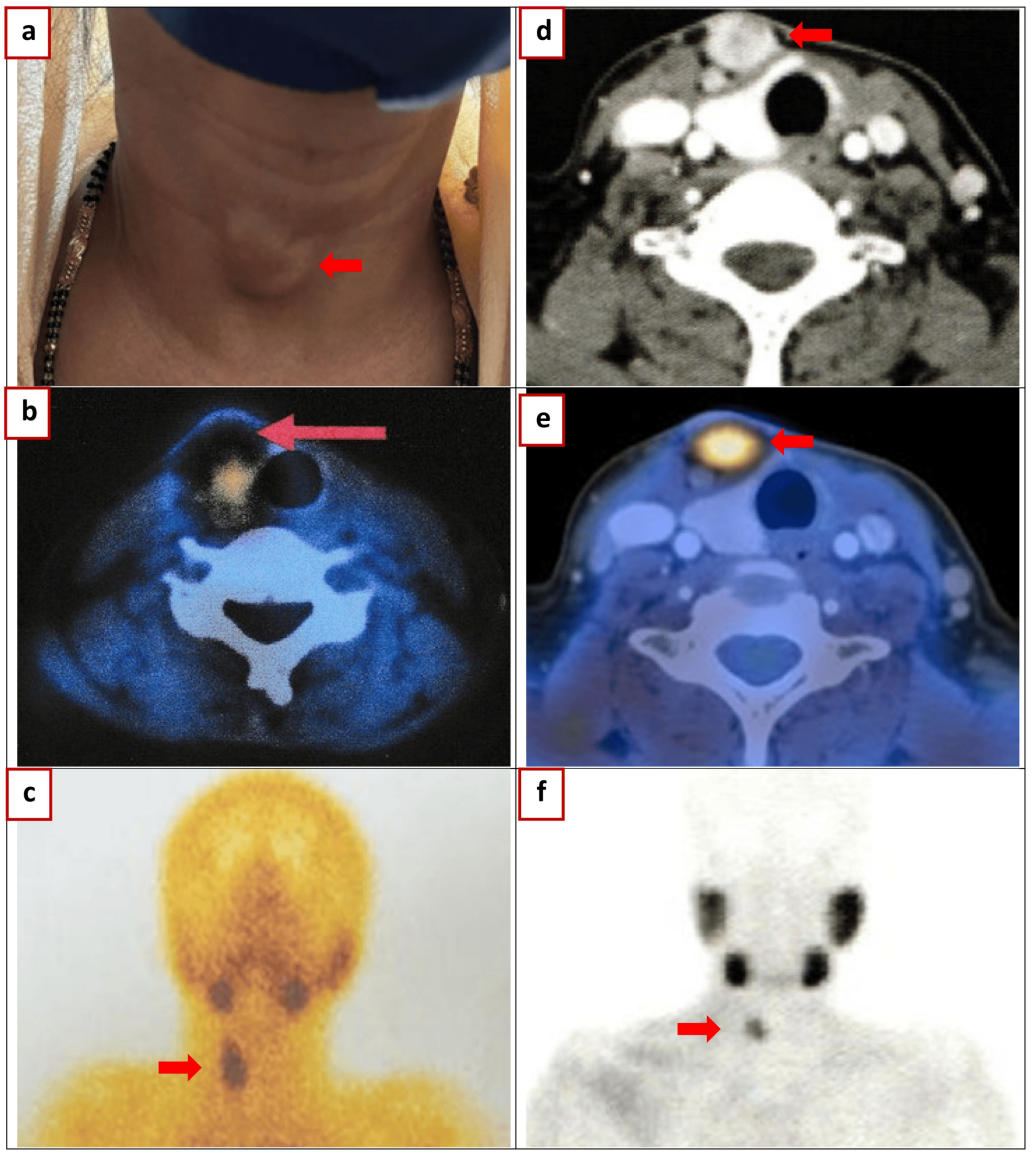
Nuclear medicine imaging (a-c) 2023 January: Technetium (Tc) 99m sestamibi single photon emission computed tomography (SPECT) CT scan: Subcutaneous cystic lesion anterior to the right lobe of the thyroid. No other evidence of abnormal focal increased MIBI uptake in the neck or mediastinum. (d-f) 2023 January: 11-Choline PET-CT: Subcutaneous nodule (17 × 15 mm) in right lower anterior neck standardized uptake value (SUV): 5.8. Left lower cervical and supraclavicular lymph nodes SUV: 2.2 (largest: 11 × 9 mm).
